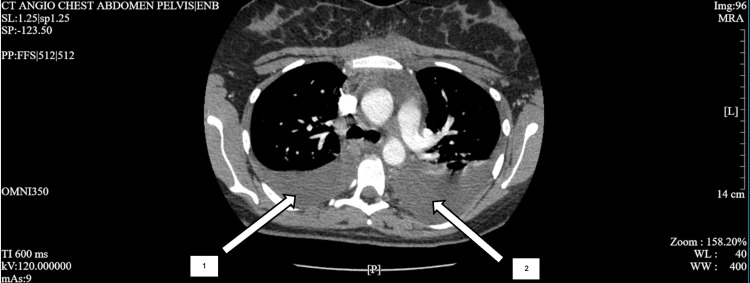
CT scan demonstrating bilateral pleural effusions taken upon admission Arrow 1 demonstrates a pleural effusion on the patient's right side and arrow 2 demonstrates a pleural effusion on the patient's left side CT: computed tomography
